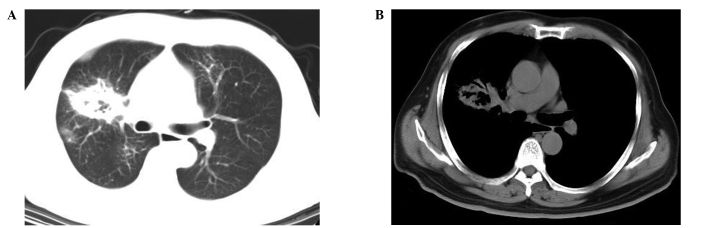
Computed tomography scan of the (A) lung window and (B) mediastinal window, revealing a mass in the right middle lobe with ground-glass opacities around it and air-filled bronchi in the consolidation.
